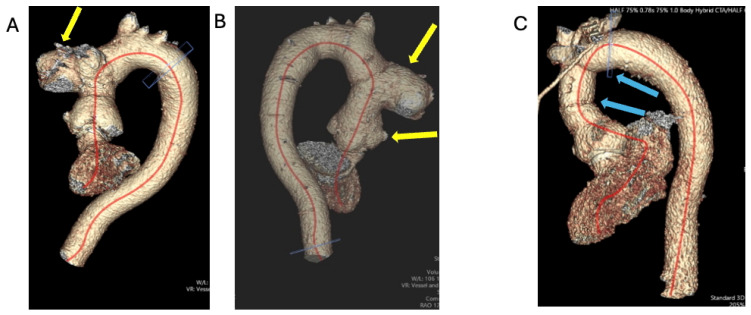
A three-dimensional rendering of CT aorta to show (A,B) the saccular pseudoaneurysm of the ascending aorta and dilated sinus of Valsalva (preoperatively, yellow arrows); (C) the replaced ascending aorta and proximal arch (post-operatively blue arrows).
